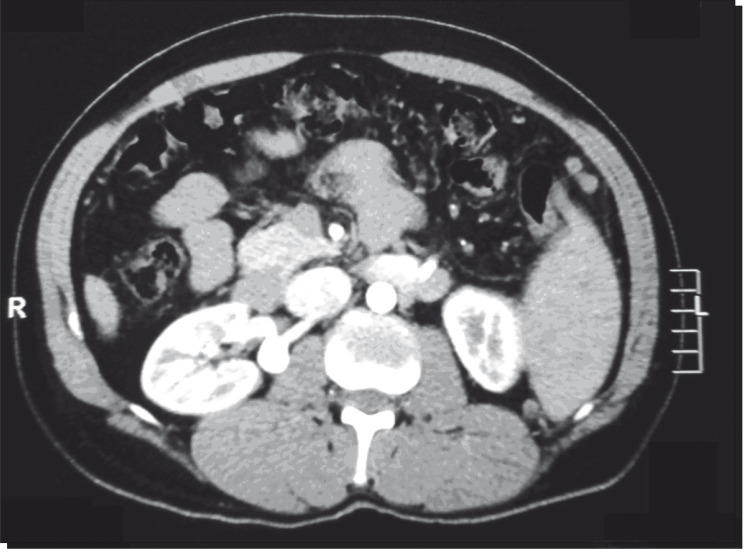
Computed tomography showing right renal artery aneurysm.
